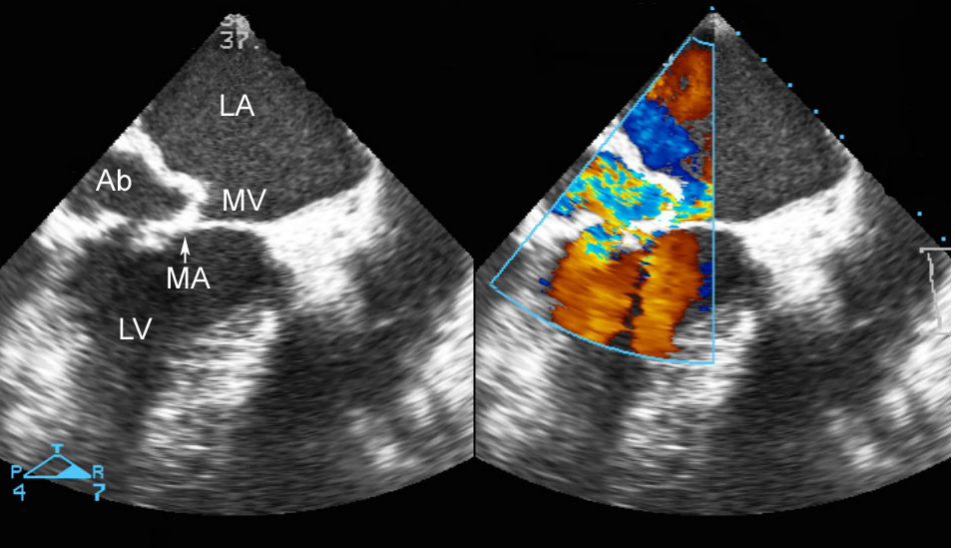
Transesophageal echocardiogram showing mitral paravalvular abscess with ventriculo-atrial fistula. LA indicates left atrium; MV, mitral valve; MA, mitral annulus; LV, left ventricle; and Ab, abscess.
